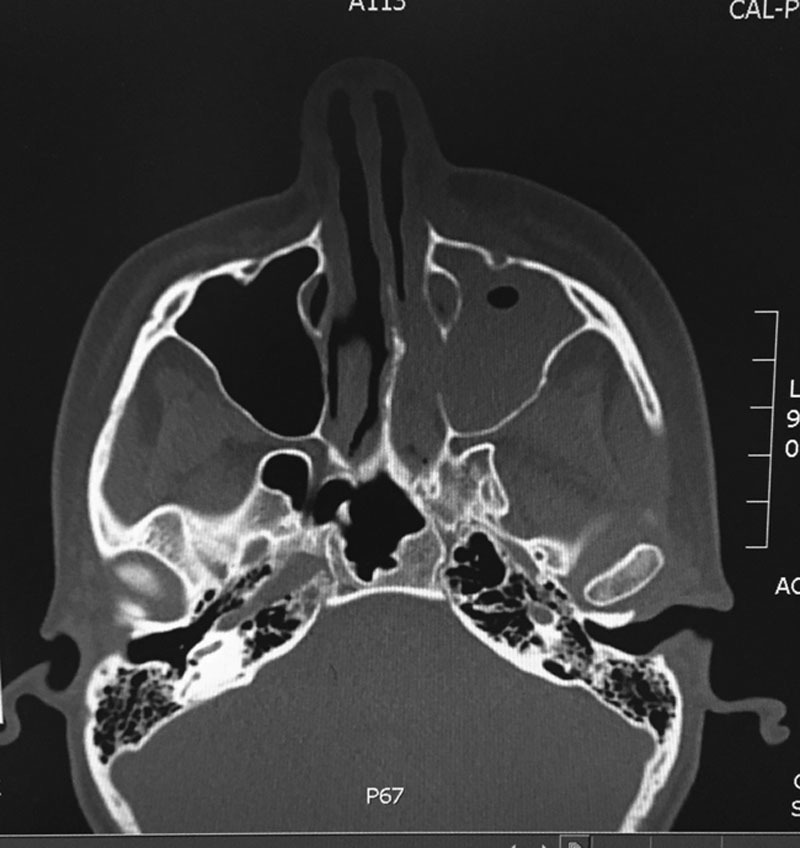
Axial view of computed tomography images demonstrating initial maxillary sinusitis.
